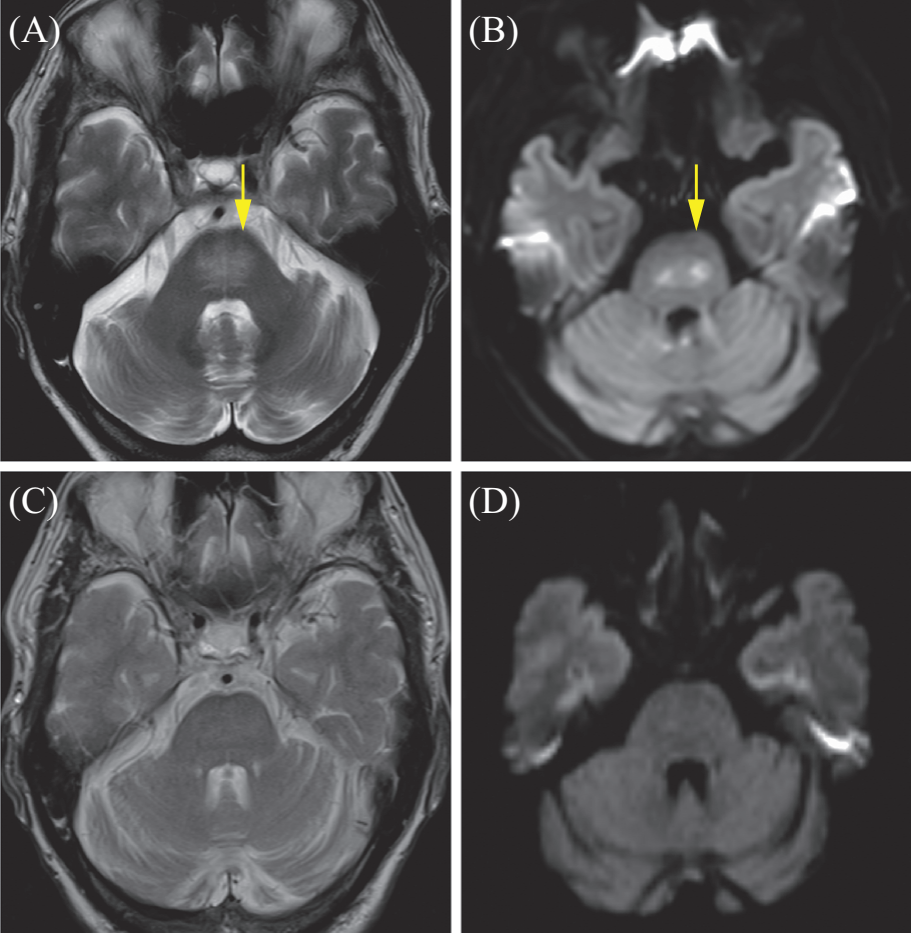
A hyper‐intense lesion in the pons (arrow) on brain magnetic resonance imaging (MRI) was observed on T2‐weighted imaging (T2WI) (A) and diffusion‐weighted imaging (dWI) (B). MRI demonstrated disappearance of the hyper‐intense lesion in the pons on T2WI (C) and dWI (D) after three cycles of R‐GDP therapy
